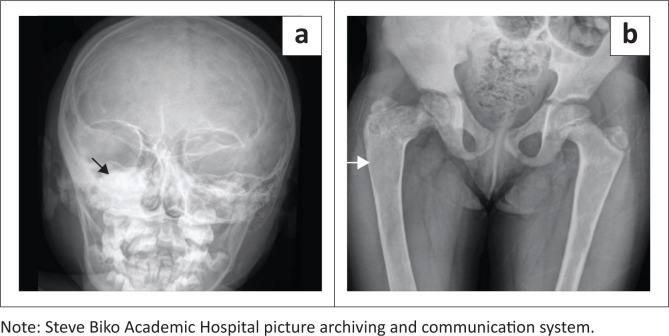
Anterior-posterior (AP) radiograph of the skull (a) demonstrates a unilateral, expansile sclerotic lesion of the spheno-temporal bone (black arrow). AP radiograph of the pelvis and proximal femora (b) demonstrate an expansile, mixed, lytic-sclerotic lesion of the right proximal femur (white arrow) with endosteal scalloping.
